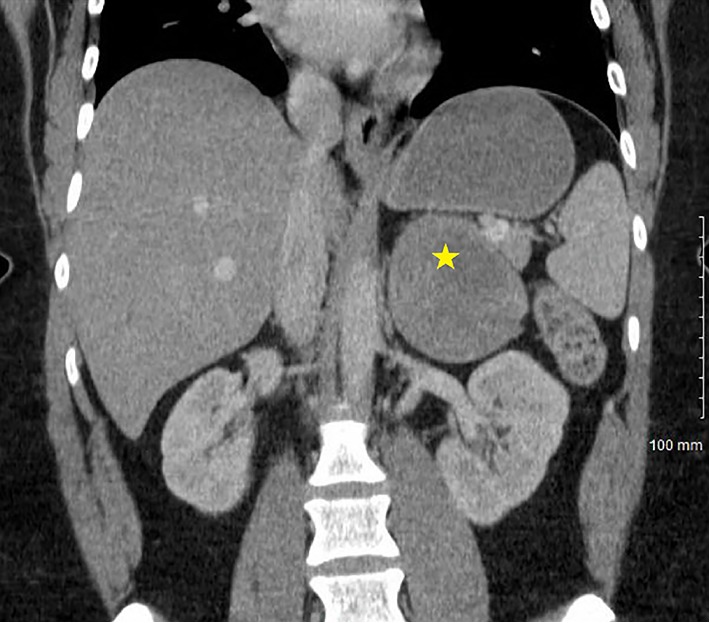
Contrast enhanced computed tomography imaging of abdomen, coronal view: left adrenal mass measuring 7.4 × 7.3 × 8.0 cm (star).
